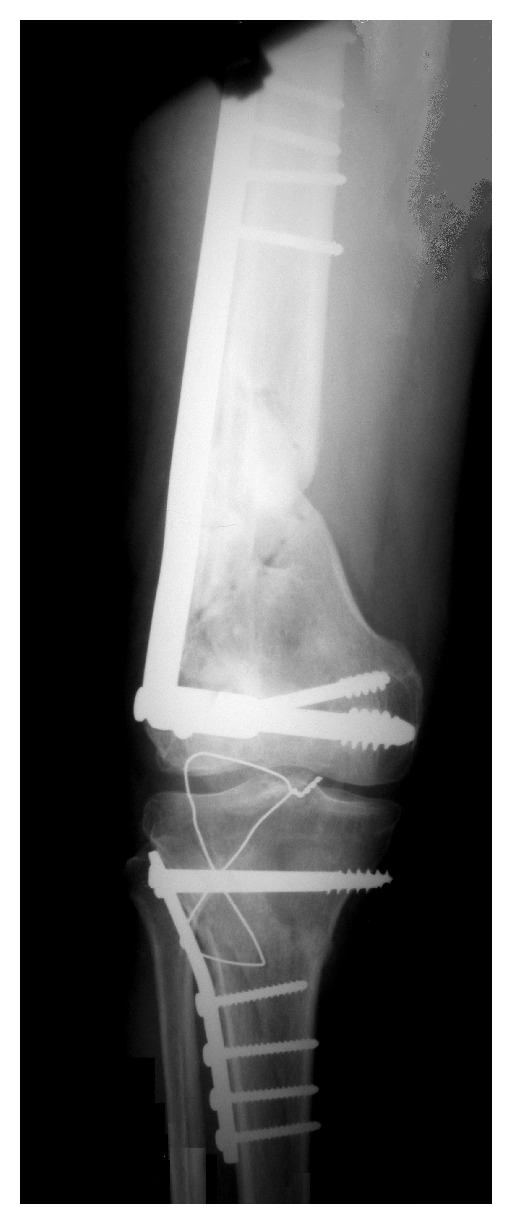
3-month followup X-ray of the right knee AP showing fracture union.
